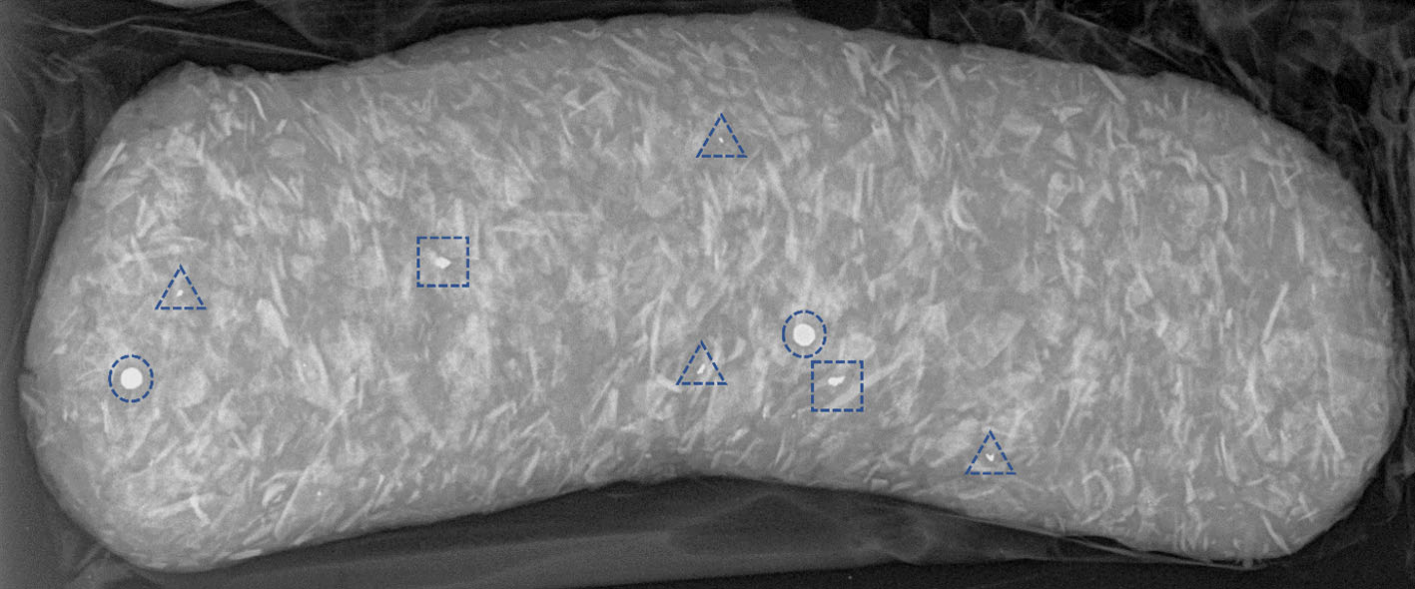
X-ray of a pack of petfood consisting of raw mince derived principally from pheasants. Spherical radio-dense objects assumed to be whole shot are indicated by circles (*n* = 2). Large radio-dense fragments are indicated by squares (*n* = 2) and small fragments by triangles (*n* = 4)
